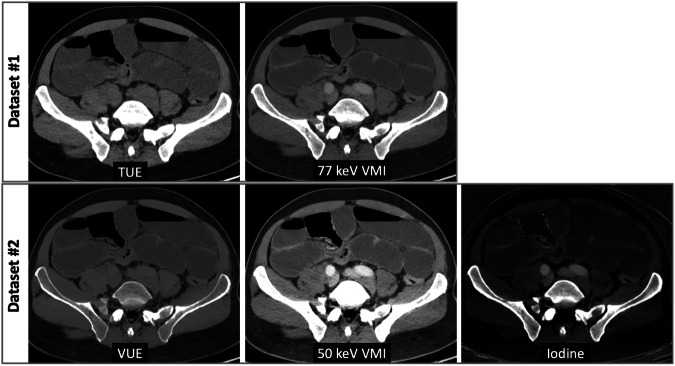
Image combination used for each dataset. The dataset#1 included the TUE images and the monoenergetic images at 77 keV, while the dataset #2 included the VUE images, the monoenergetic images at 50 keV, and the iodine images. Hence, datasets #1 and #2 corresponded to 120 kVp-equivalent polychromatic CT examination and DECT examination, respectively. TUE, true unenhanced; VUE, virtual unenhanced; VMI, virtual monoenergetic image
